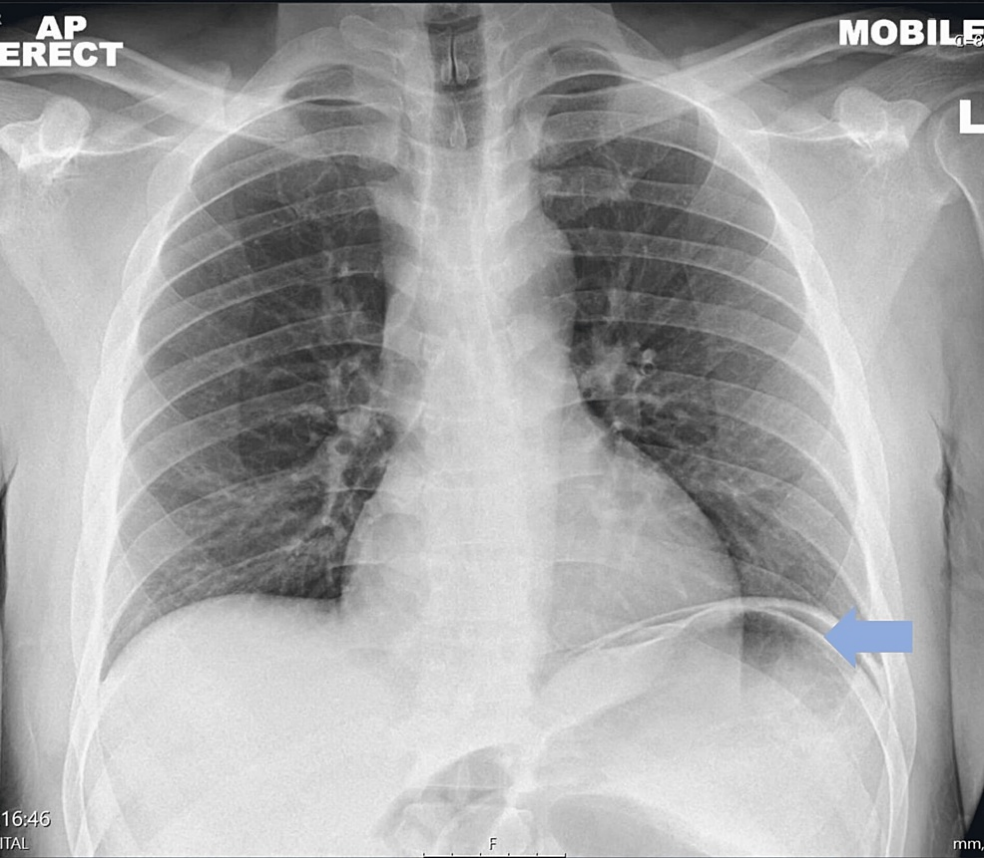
Chest radiography demonstrating air under the diaphragm highlighted by the colored arrow.
